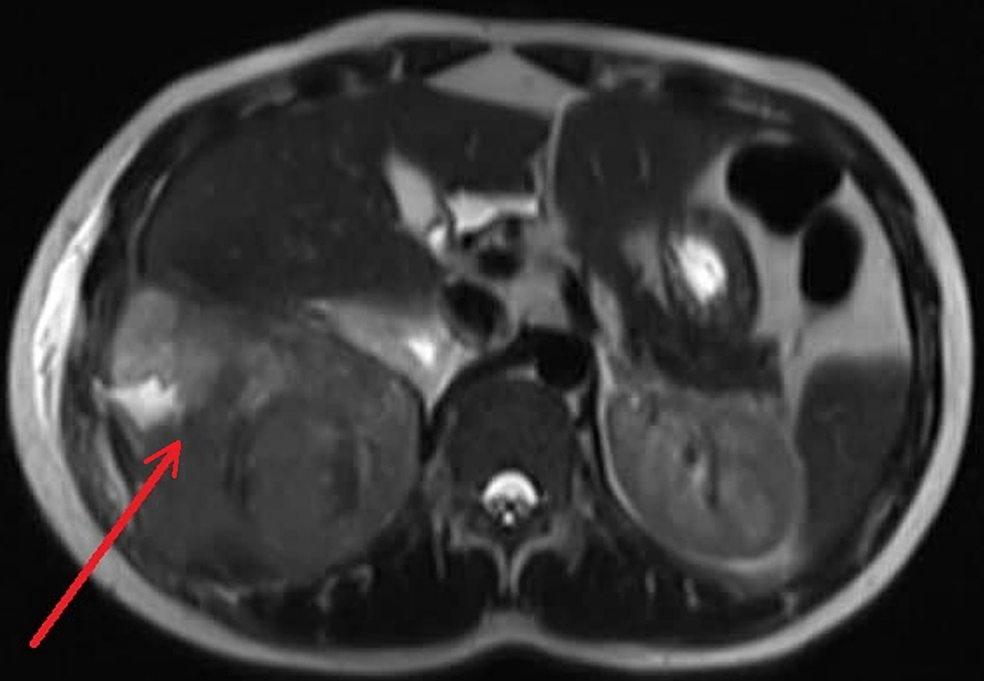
MRI of the abdomen in T2 shows a predominantly solid heterogeneous mass lesion (red arrow) noted in relation to the right lower posterolateral chest wall above and below the diaphragm with hyperintense areas that are suggestive of cystic changes toward the peripheral part.
